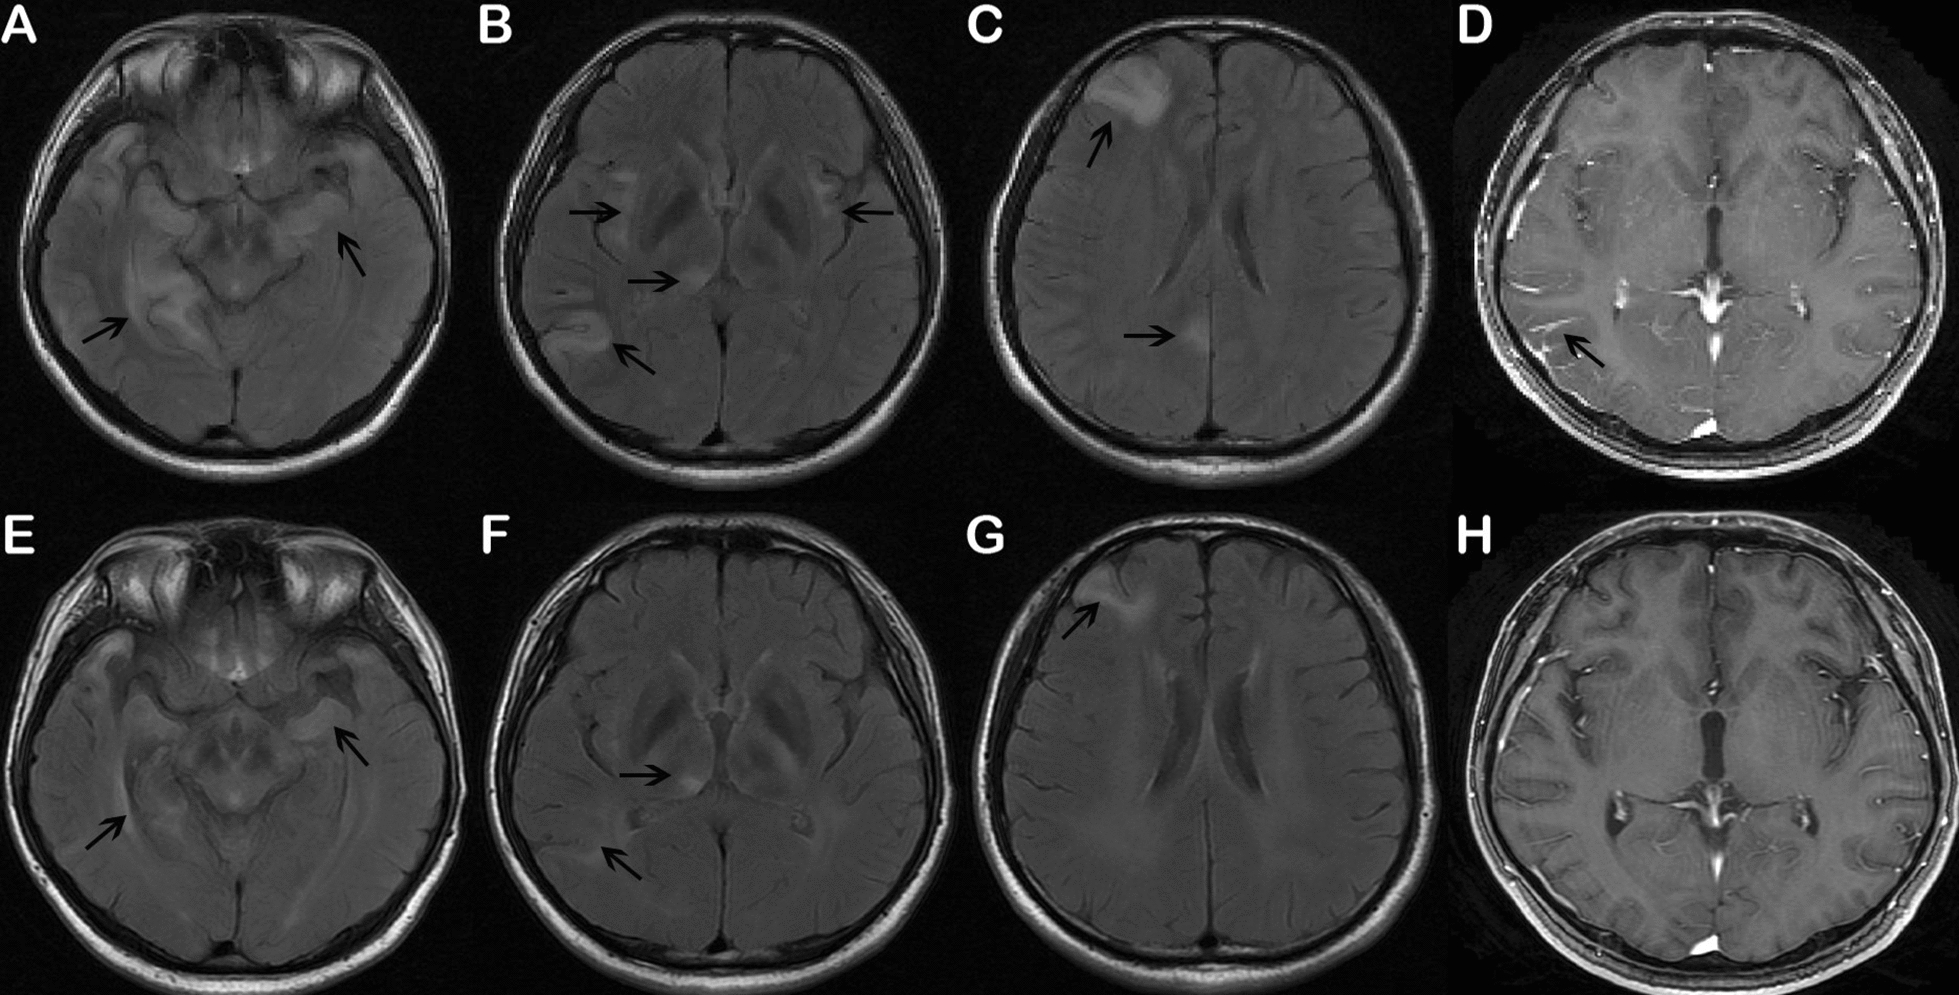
Initial and follow-up brain MRI findings. A–C Axial FLAIR images showed hyperintensities in bilateral medial and anterior temporal lobes, insula, right pulvinar of the thalami, precuneus, frontal and temporo-occipital lobes. D T1-contrast-enhanced MRI showed leptomeningeal enhancement in the right temporo-occipital region. E–G Post-treatment FLAIR images on 16th day of admission revealed marked improvement in the previously identified bilateral temporal, insula, precuneus, frontal and temporo-occipital hyperintensities. H Repeat gadolinium-enhanced MRI demonstrated disappearance of leptomeningeal contrast enhancement. The arrows indicated the lesions
